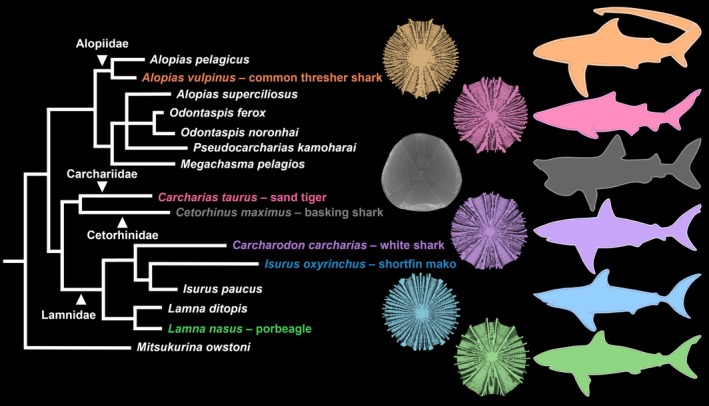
Phylogenetic tree of lamniform sharks. Species examined in this study are listed in color with common names. A transverse model of a centrum was created for each species via the *Segmentation Editor* module in 3D Slicer and is shown next to an outline of the respective species. A transverse section of the micro‐CT scan for the basking shark is shown due to the poor mineralization and difficulty of creating a segmentation. Tree adapted from (Naylor et al., 2012).
